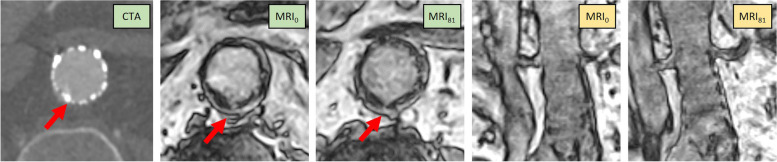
Transverse (green) and coronal (yellow) views of patient 2. The red arrows are pointing at a location of no circumferential apposition that becomes better visible on upright MRI compared to supine imaging. *MRI*, Magnetic resonance imaging
